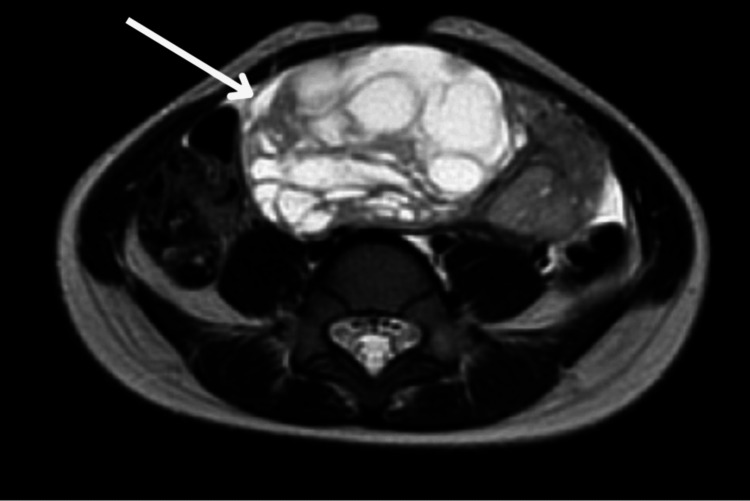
Axial T2-weighted MRI image demonstrating a well-defined pelvic mass (arrow) with hyperintense solid and cystic components, suggestive of a JGCT JGCT: juvenile granulosa cell tumor; MRI: magnetic resonance imaging
